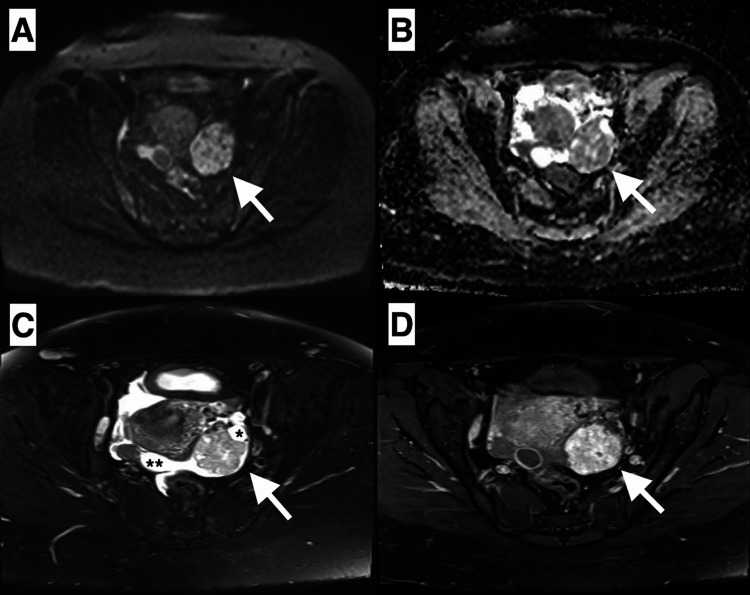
Diffusion restriction in solid component of the left adnexal mass revealed on the diffusion-weighted image and apparent diffusion coefficient mapping (A,B). A heterogeneous mass (arrow) with solid and cystic (*) components, pelvic free fluid () were revealed on fat-sat T2-weighted images (C). After gadolinium administration, fat-sat T1-weighted image revealed heterogeneous intense enhancement in the solid component of the left adnexal mass (D).
